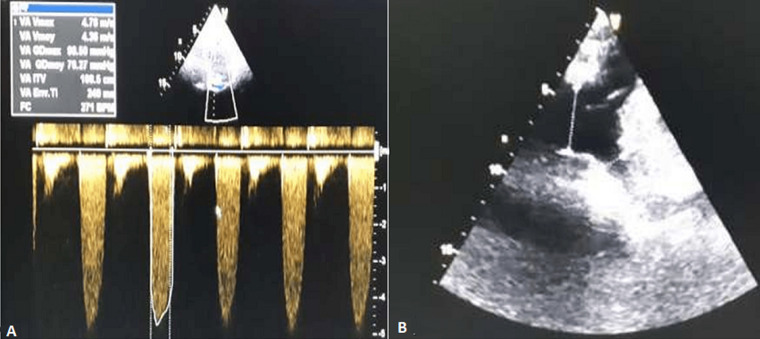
continuous aortic Doppler, A) showing the peak transvalvular pressure gradient and mean transvalvular pressure gradient respectively of 164/74 mmHg, the sub sternal incidence; B) showed an enlarged ascending aorta and subclavian artery
